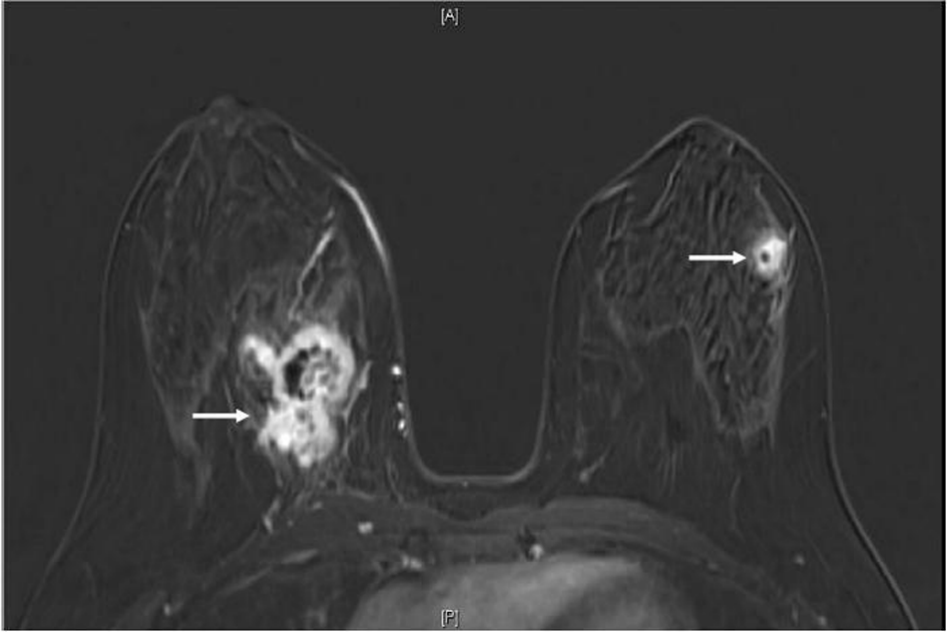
MRI breast (T1, fat saturated, post-contrast subtraction image) showing the largest right breast mass (left arrow) which is heterogeneously enhancing, with central necrosis and the largest left breast mass (right arrow), also heterogeneously enhancing with central necrosis.
